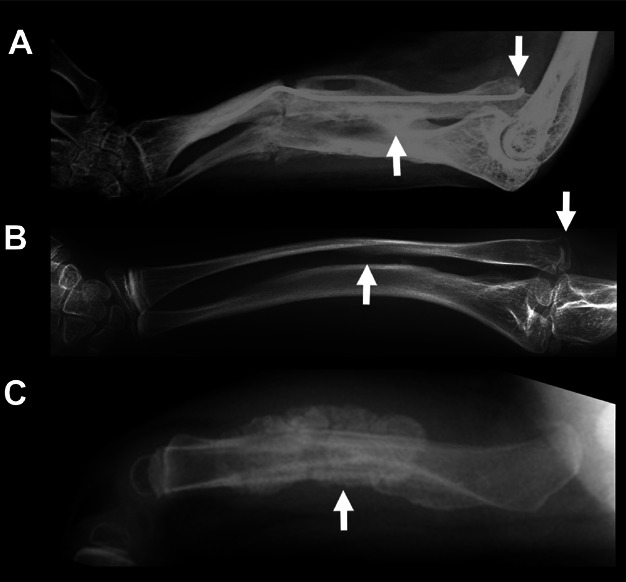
Radiographic features in OI type V. (*A*, *B*) Radial head dislocation (arrows on the right) and forearm interosseous membrane calcification (middle arrows) in patients 1 and 3, respectively. (*C*) HPC in patient 14 at 1.5 years.
